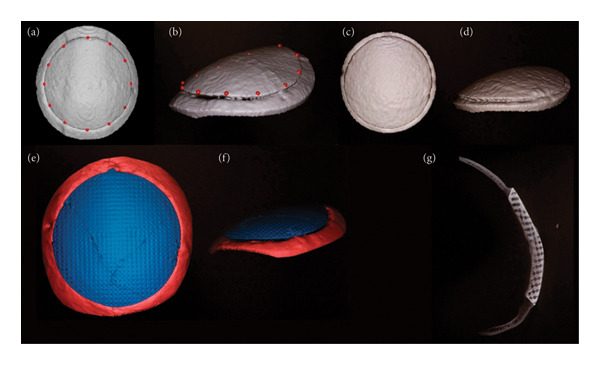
Longitudinal CT and postmortem CT analysis. By applying thresholds for bone (250–3070 HU), 3D models of the cranioplasty and surrounding bone were created. (a) Marking models in a clockwise pattern divided the cranioplasty’s perimeter into 12 distinct regions. Two independent raters scored these regions for the degree of bridging. (b) Bridging percentage was described as the presence of approximation between the cranioplasty and native bone. (c and d) While the final longitudinal CT obtained 12 months postoperative was used to measure the presence of approximation, (e and f) micro‐CT created a higher resolution model, which could assess integration. (e) After obtaining micro‐CT, a single instance of subclinical implant fracture was observed. Following fracture, the implant remained in place and the subject showed no clinical signs of distress. These high‐resolution models were able to evaluate bony ingrowth within the implant by measuring differences in volume. (g) Mid‐sagittal slice of a BMP sample demonstrating bony union/integration with adjacent skull. To assess the degree of integration, equally spaced landmarks were placed on the 3D reconstructions around the periphery of the interface (see Table 2).
